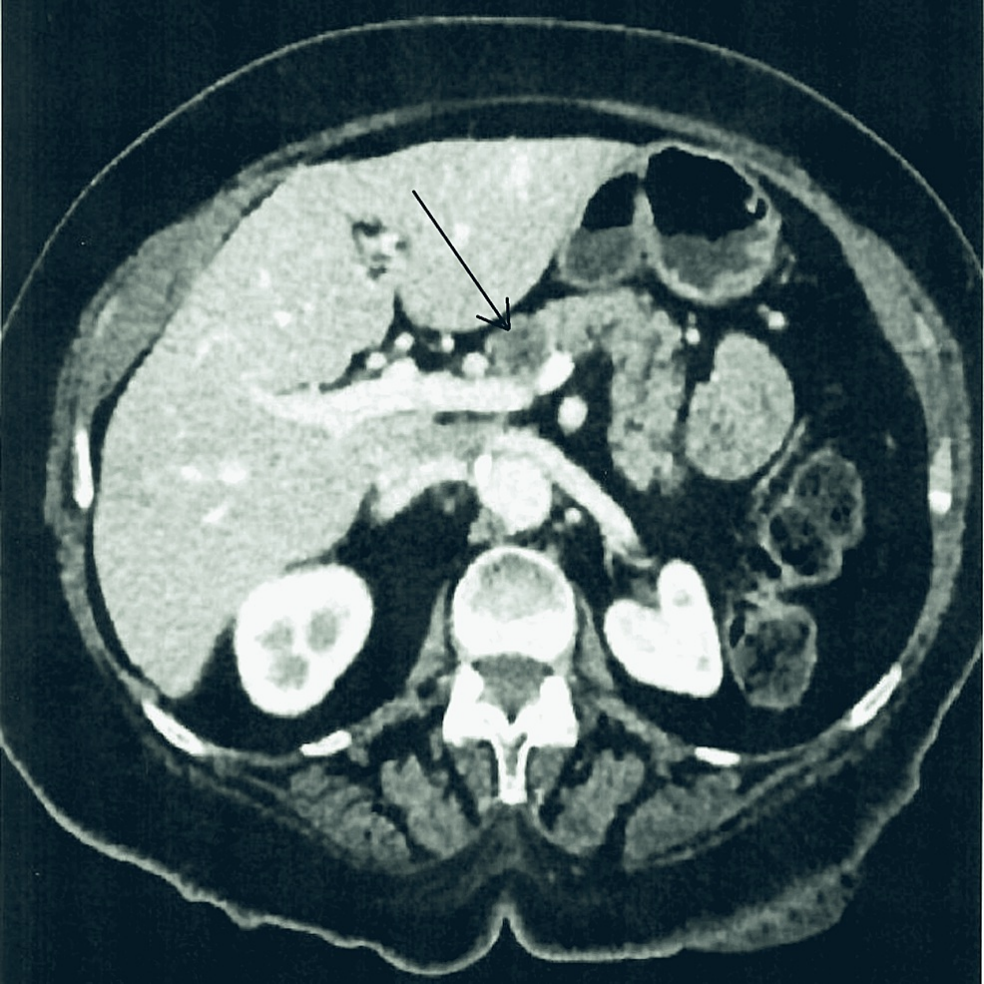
Pancreatic schwannoma on computed tomography scan of the abdomen (arrow).
